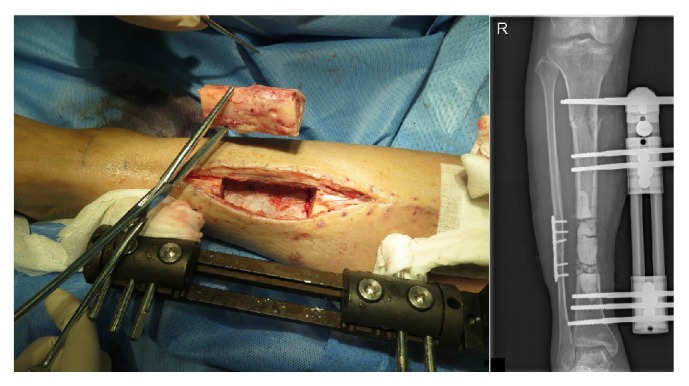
The left figure was the resection of infected bone segment when radical debridement was conducted. The right figure was the anteroposterior X-ray photograph taken few hours after surgery, and antibiotic-impregnated calcium sulphate was demonstrated to be filled in the bone defect.
